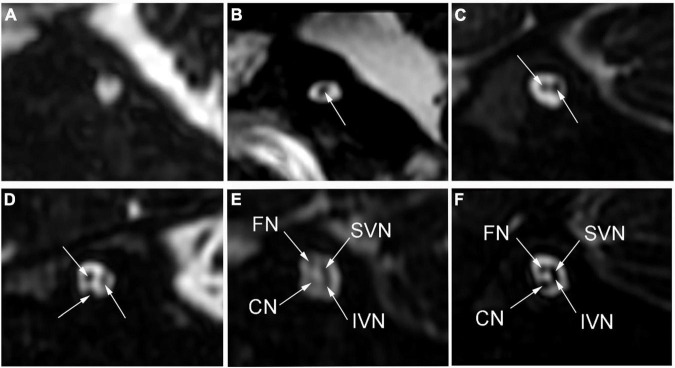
The number of nerve bundles (indicated by the white arrows) in the internal auditory canal on oblique sagittal magnetic resonance imaging. (A) No nerve bundle, (B) one nerve bundle, (C) two nerve bundles, (D) three nerve bundles, (E) four nerve bundles (thin), (F) four nerve bundles (normal); CN: cochlear nerve, FN: facial nerve, IVN: inferior vestibular nerve, SVN: superior vestibular nerve.
